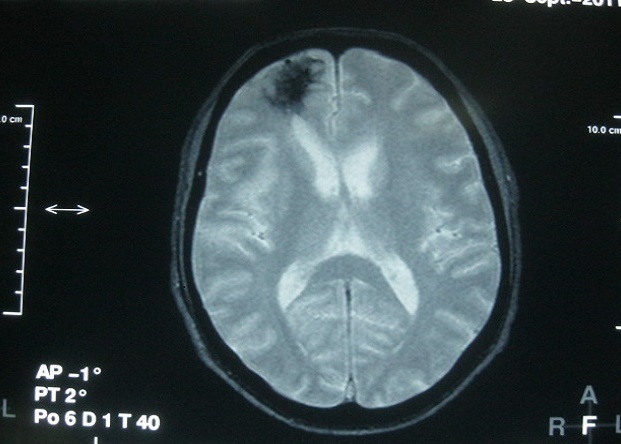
Brain MRI showing hypointensity of the right fronto-polar region on T2^*^ image
